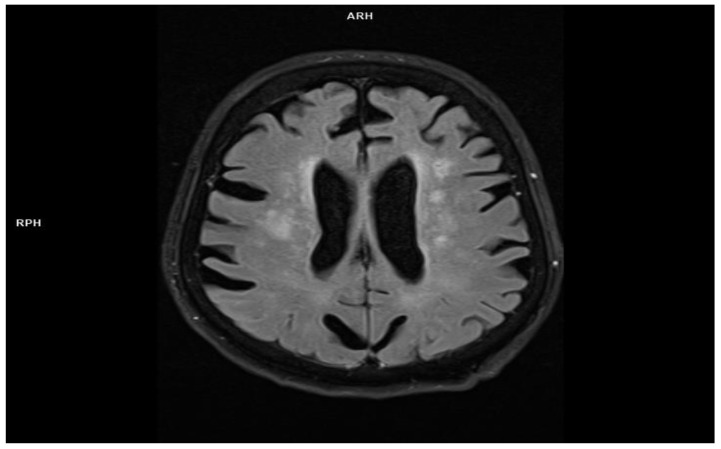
Cortical-subcortical atrophy with the diffuse hyperintense areas of the white matter (small vessel disease)—Flair sequence (MRI imaging).
